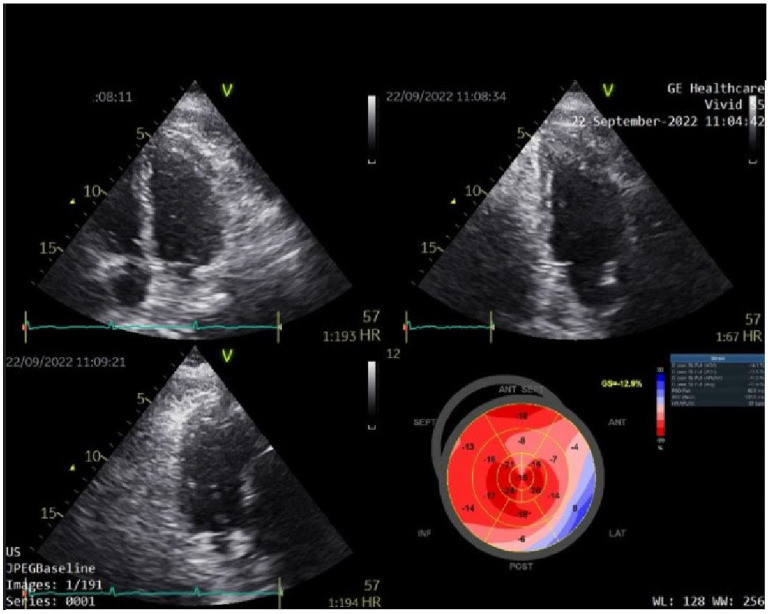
Two-dimensional echocardiography and Bull’s-eye map representation of global longitudinal strain of the left ventricle.
